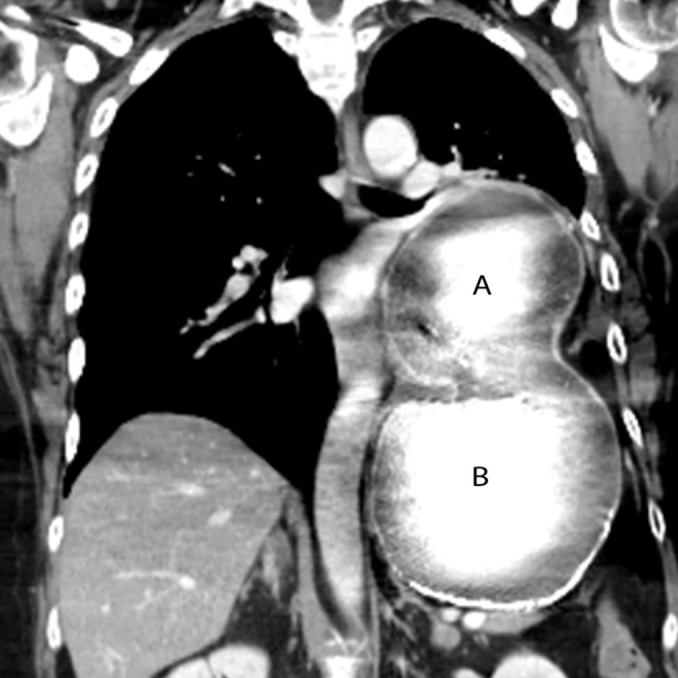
Mesenteroaxial volvulus was seen on a coronal CT of the chest and abdomen where the antrum (A) has migrated above the left diaphragm and is cephalad to the gastric body (B). *CT,* computed tomography
